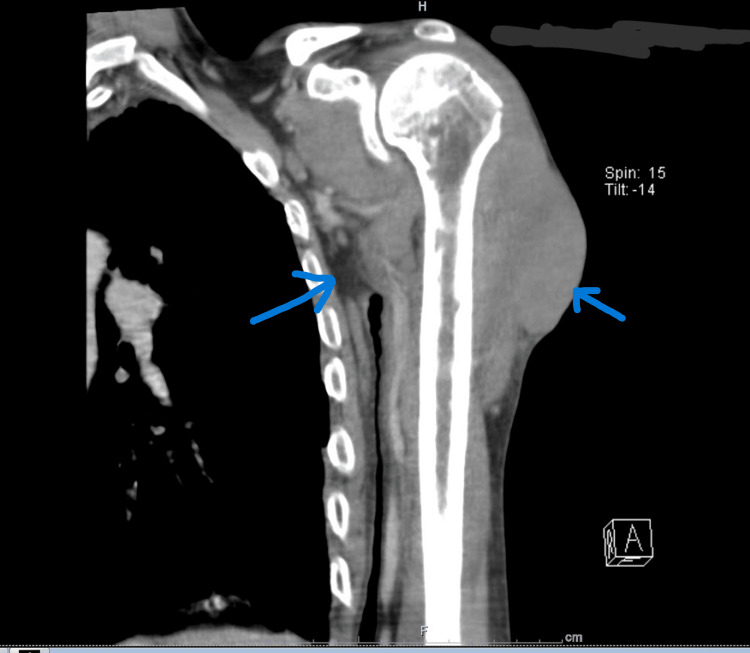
Coronal CT image demonstrating the extent of the lesion and associated regional involvement.
